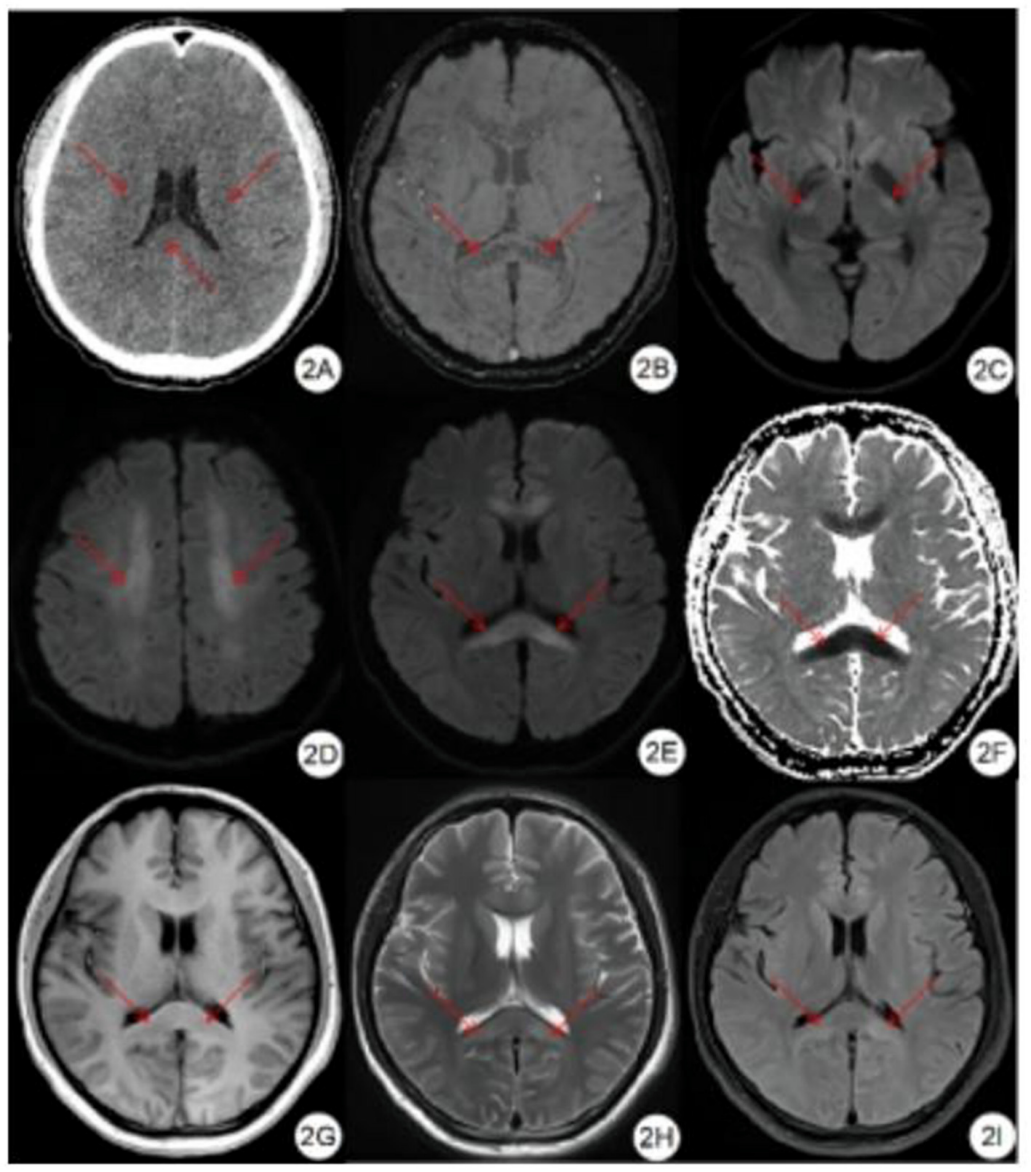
Severe HACE patient. (A) CT plain scan showed decreased density of bilateral corona radiata and corpus callosum, and shallow sulci and fission. (B) SWI showed symmetrical diffuse speckled hypointensity in the brain parenchyma, especially in the splenium and genu of the corpus callosum. (C–E) DWI showed symmetrical hyperintensity in bilateral corticospinal tract, centrum semiovale, splenium and genu of the corpus callosum. (F) ADC showed symmetrical hypointensity in the splenium and genu of the corpus callosum. (G) T1WI showed symmetrical hypointensity in the splenium and genu of the corpus callosum. (H) T2WI showed symmetrical hyperintensity in the splenium and genu of the corpus callosum. (I) FLAIR showed symmetrical hyperintensity in the splenium and genu of the corpus callosum.
